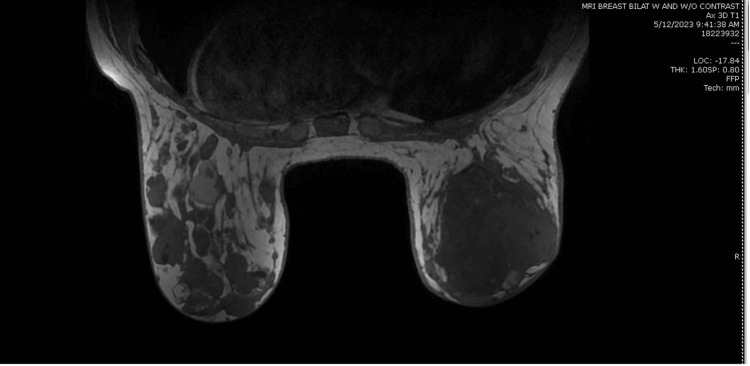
Breast MRI image The image is showing the right-sided lesion measuring 10.9 cm in greatest dimension with overlying skin thickening and enhancement.
